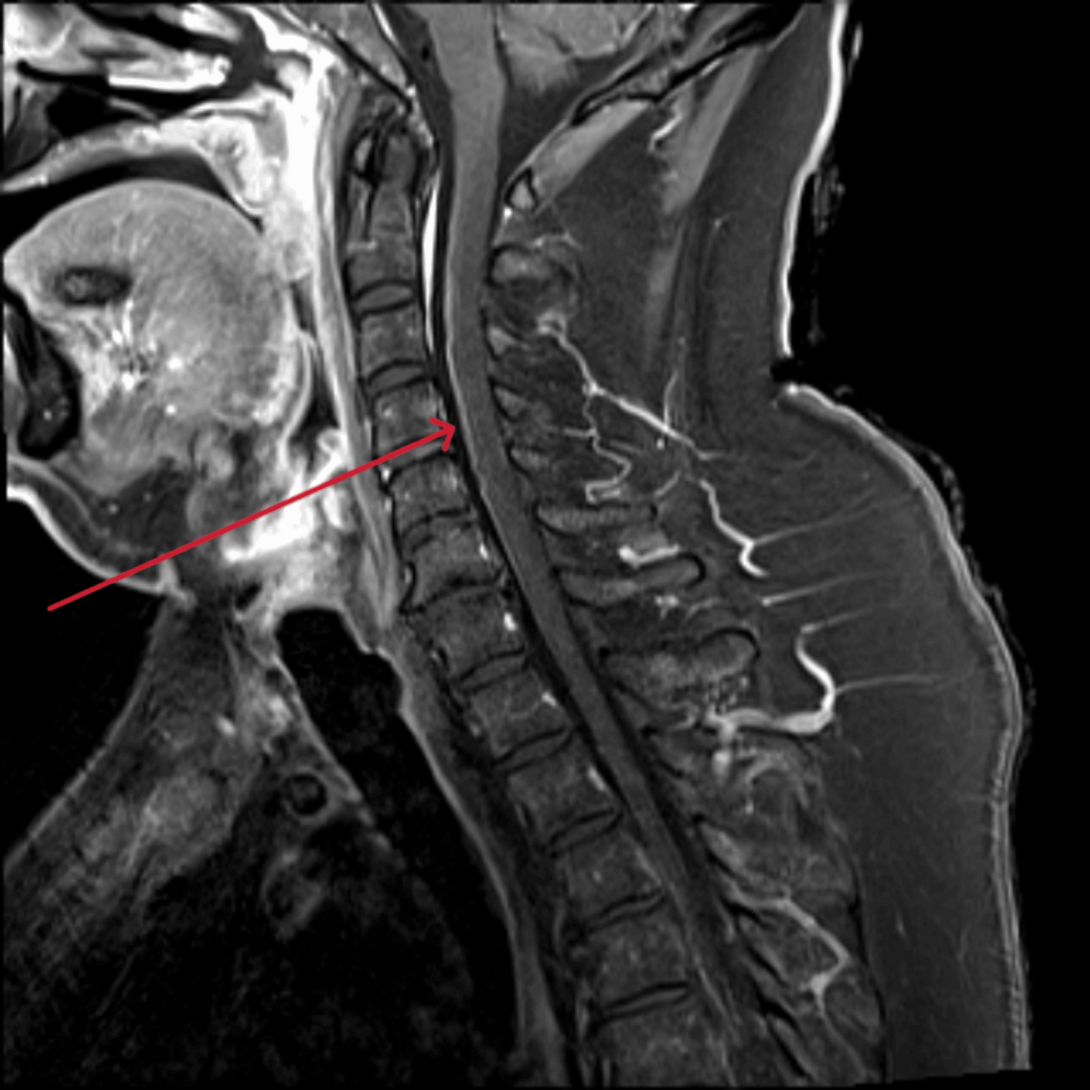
MRI showing abnormal enhancement of ventral rootlets in the cervical spine MRI: Magnetic resonance imaging
